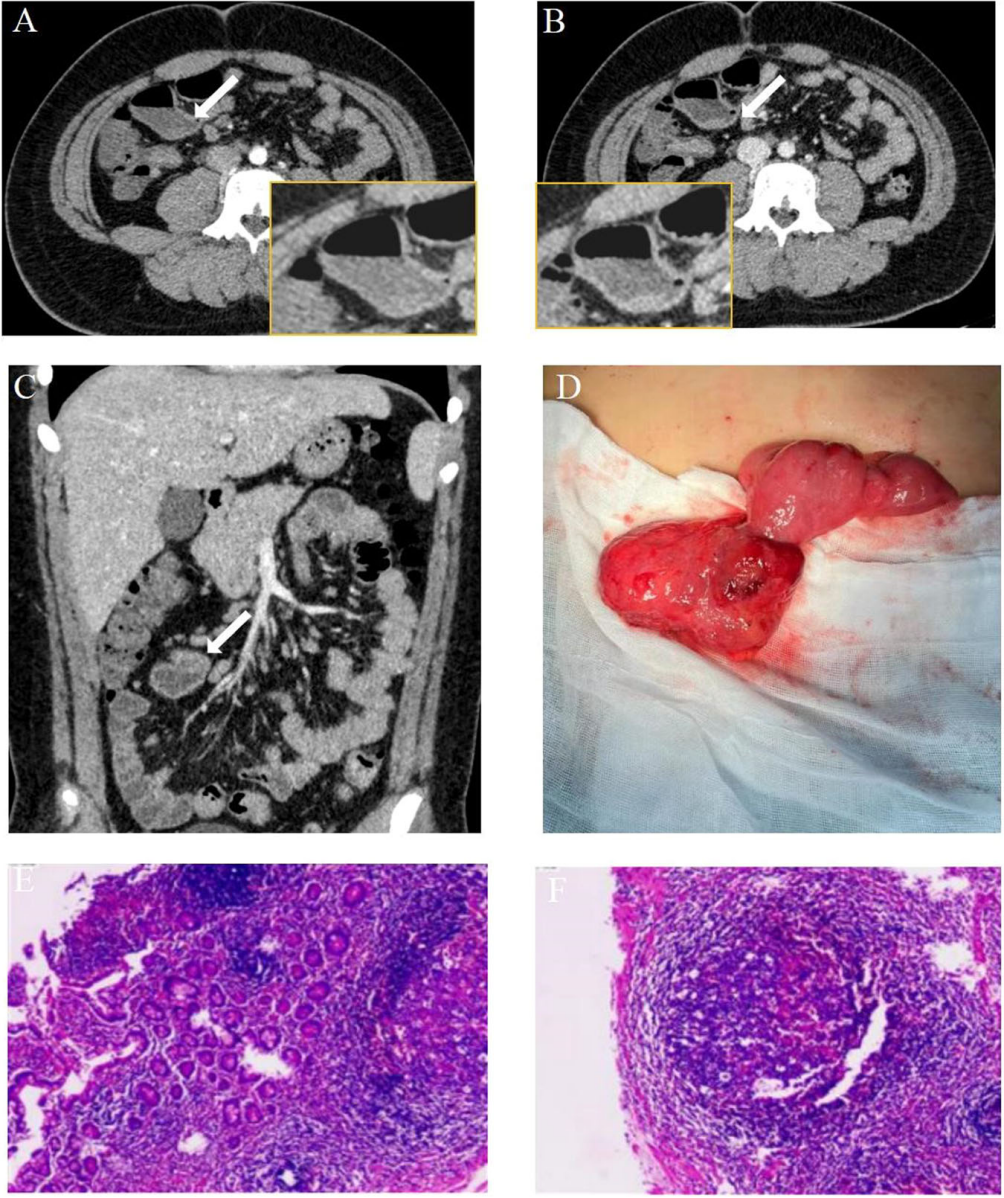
A 12-year-old boy was admitted due to profuse rectal bleeding. One day after admission, an enhanced CT scan was performed. The CT images revealed a sac-like structure with a minimal accumulation of contrast material during the arterial (A) and venous (B) phases. The CT diagnosis was Meckel’s diverticulum bleeding. C Depicts the Meckel’s diverticulum on the coronal plane (white arrow). Ten days later, D surgery confirmed the presence of diverticular bleeding located 25 cm from the ileocecal valve, and pathological results (E, F) indicated Meckel’s diverticulum (HE × 100, × 200)
